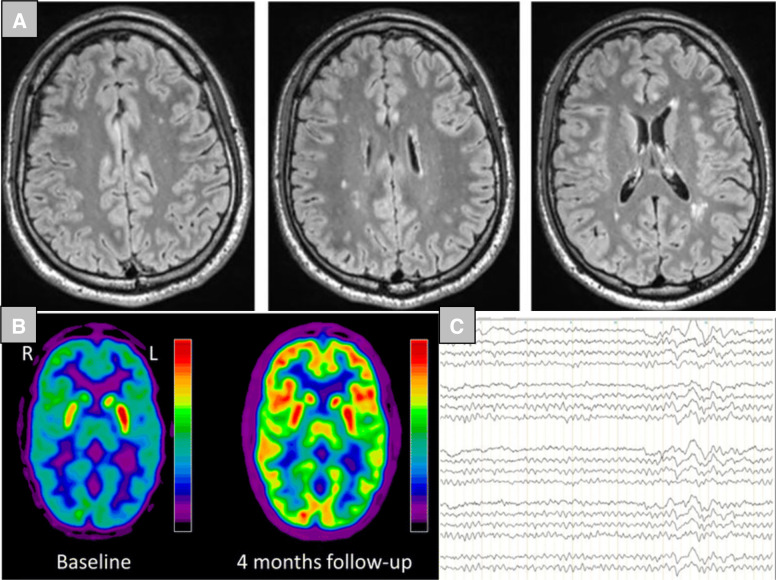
Exemplary findings suggestive of an autoimmune cause of obsessive-compulsive symptoms. A The magnetic resonance imaging (MRI) shows multiple chronic inflammatory white matter lesions using FLAIR sequences. The corresponding patient was 22 years old at the time of the MRI, was diagnosed as neuropsychiatric lupus erythematosus and suffered from an atypical OCD with abrupt onset and additional psychotic symptoms. Immunotherapy with steroids, methotrexate and hydroxychloroquine resulted in an impressive clinical improvement [46] (online available at: https://www.frontiersin.org/articles/10.3389/fpsyt.2019.00226/full, retrieved on 30 January 2021; the reproduction was allowed by the publisher according to the Creative Commons Attribution License [CC BY]). B The [^18F^]fluorodeoxyglucose positron emission tomography (FDG-PET) findings are from of an 18-year-old male patient with suspected PANDAS syndrome. Baseline images showed moderate to severe hypermetabolism of the left striatum. The cortex showed a hypometabolic signal. The metabolic changes disappeared completely after plasmapheresis treatment at 4 months follow-up [67] (online available at: https://bmcneurol.biomedcentral.com/articles/10.1186/s12883-018-1063-y, retrieved on 30 January 2021; the reproduction was allowed by the publisher according to the Creative Commons Attribution 4.0 International License). C Intermittent rhythmic generalized electroencephalography (EEG) slowing in 41-year-old female patient with Hashimoto encephalopathy [129] (online available at: https://www.frontiersin.org/articles/10.3389/fpsyt.2017.00064/full, retrieved on 30 January 2021; the reproduction was allowed by the publisher according to the Creative Commons Attribution License [CC BY]). This EEG phenomenon is frequently found in patients with autoimmune psychiatric syndromes [75].
